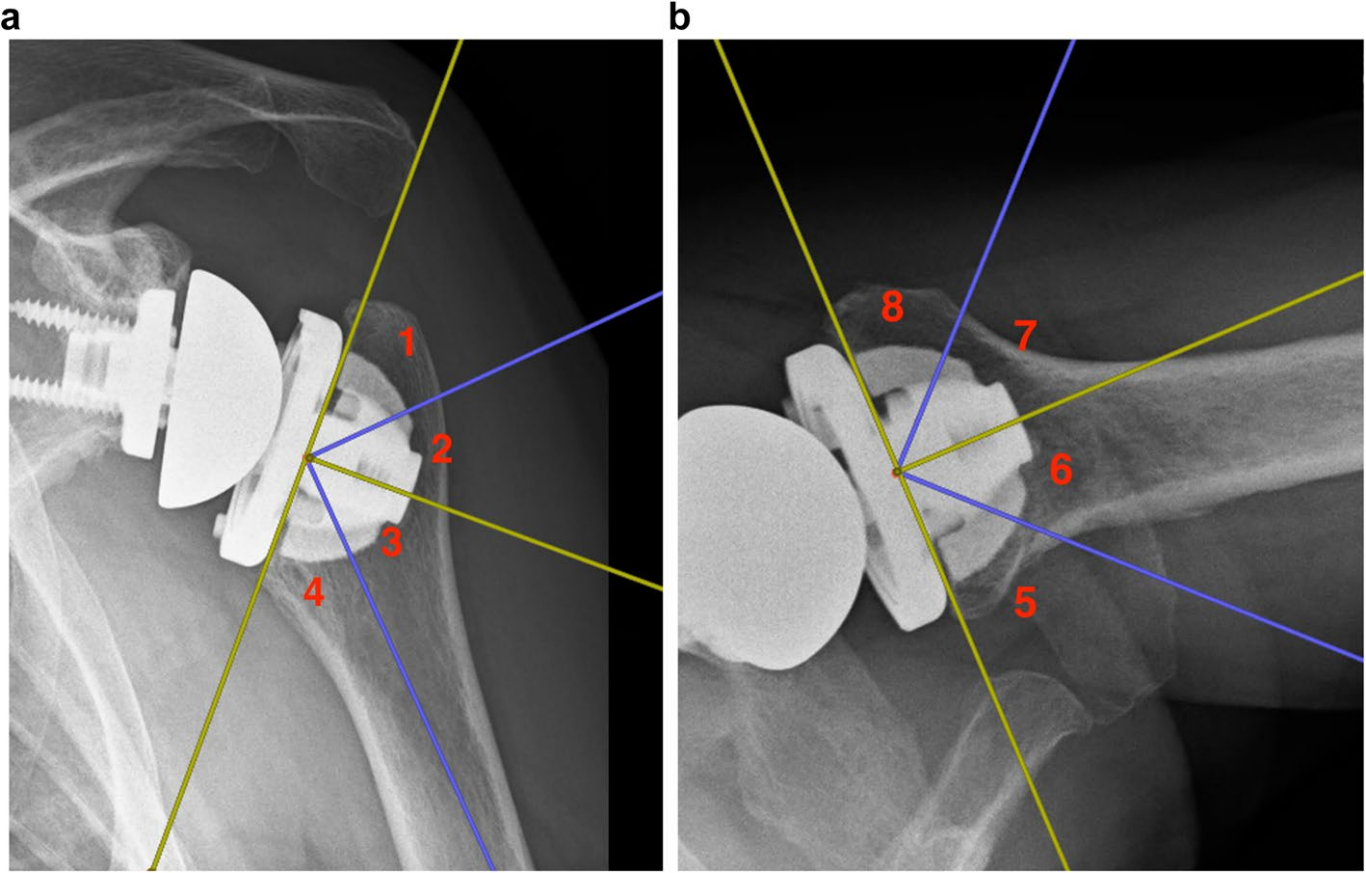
For radiological assessment, the humerus was divided into 8 distinct zones on antero-posterior (zones 1--4) and axillary (zones 5--8) X-rays according to Moroder et al. [20]
